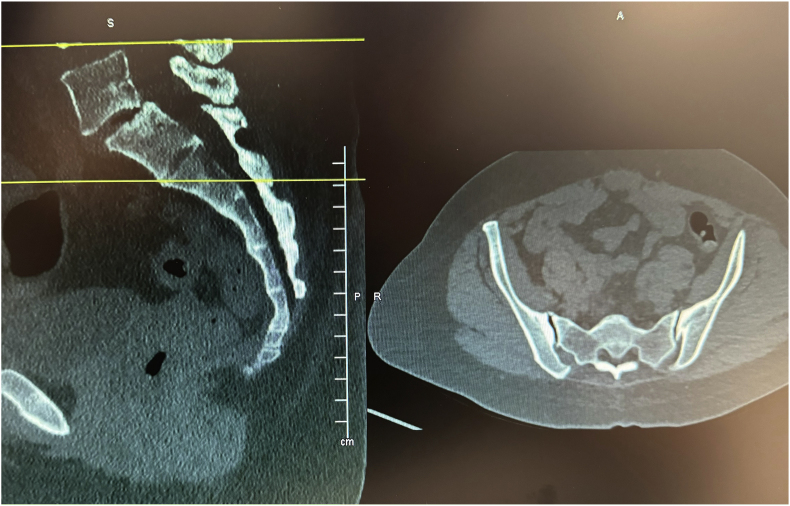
Accessory SIJ and varus angulation with S-curvature. This figure depicts a CT image where accessory SIJ and varus angulation with S-curvature were identified during pre-operative mapping.
